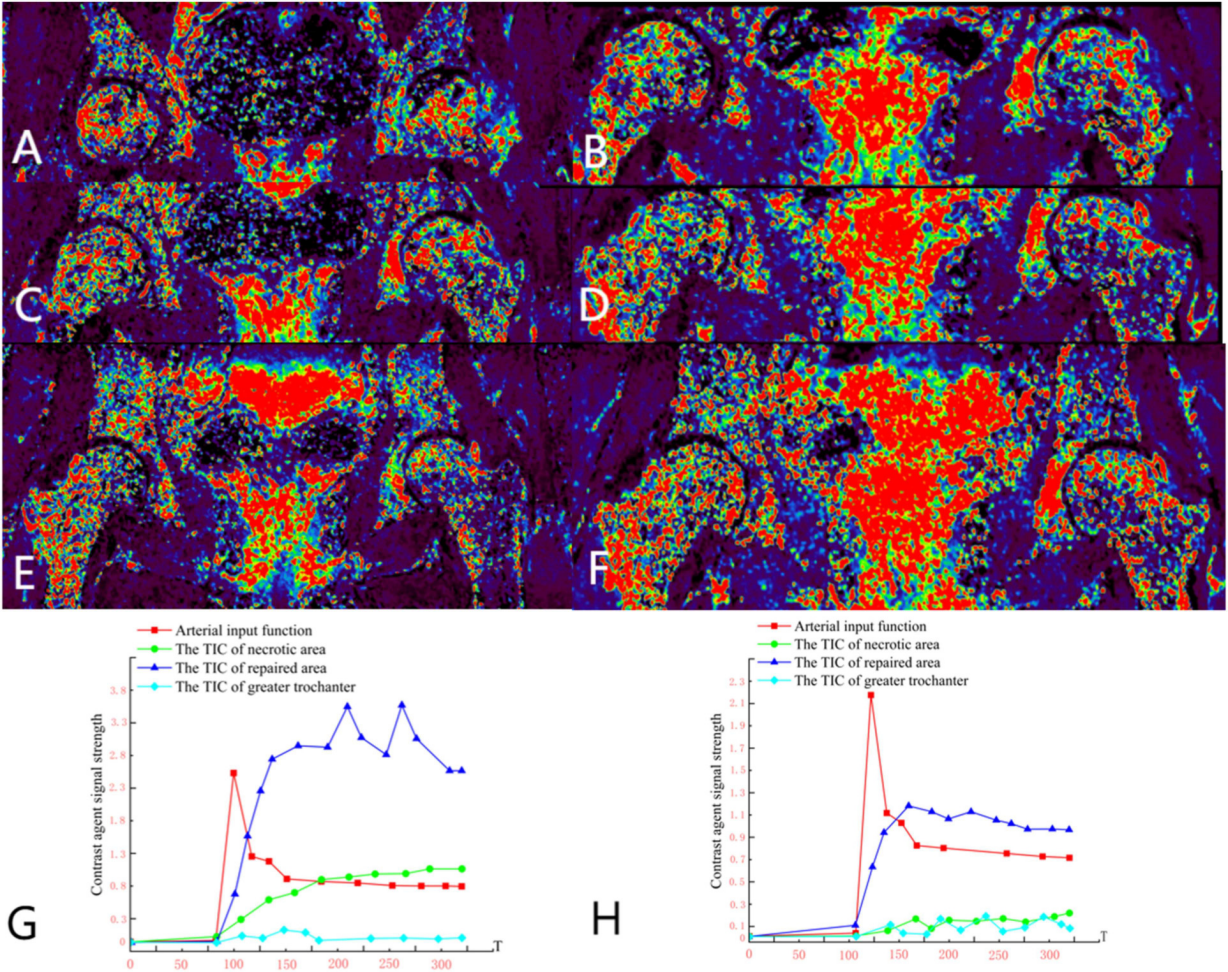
(A,C,E) Preoperative DCE-MRI Ktrans pseudocolor maps; (B,D,F) postoperative; (G) preoperative TIC curve; (H) postoperative TIC curve. Preoperative DCE-MRI indicates poor blood perfusion in the right femoral head, with internal instability and a high risk of collapse. The postoperative DCE-MRI shows that compared to the preoperative images, not only the bone marrow edema in the femoral head (sclerotic area) diminished, but also necrotic area shown signs of revascularization.
